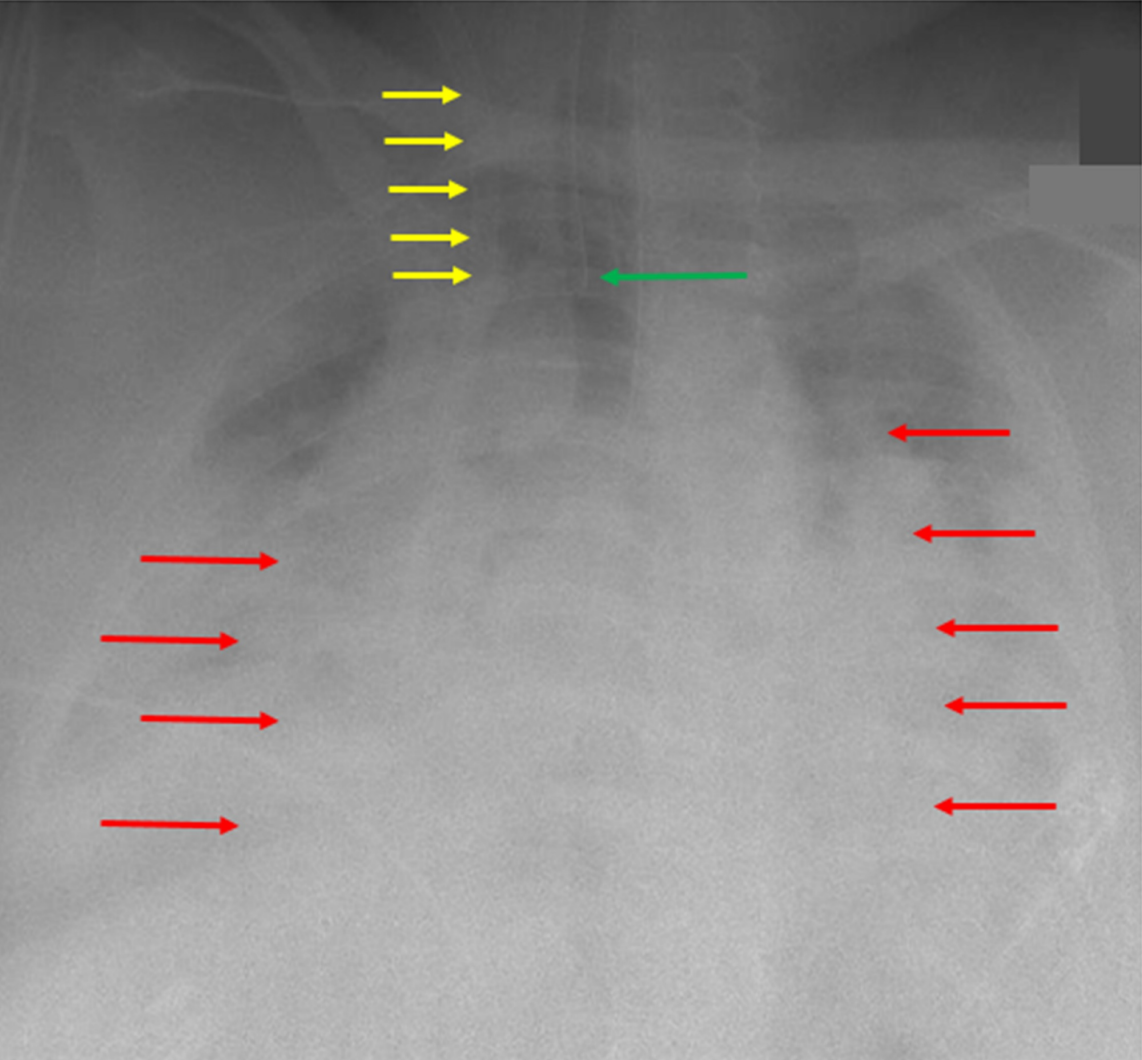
Follow-up radiograph after adjustment of the extracorporeal membrane oxygenation catheter A chest radiograph is shown after adjustment of the extracorporeal membrane oxygenation catheter (yellow arrows) in the right atrium of the heart. There is worsening bilateral pulmonary opacities (red arrows) present as a result of acute respiratory distress syndrome. The endotracheal tube (green arrow) can be seen appropriately placed just above the carina.
